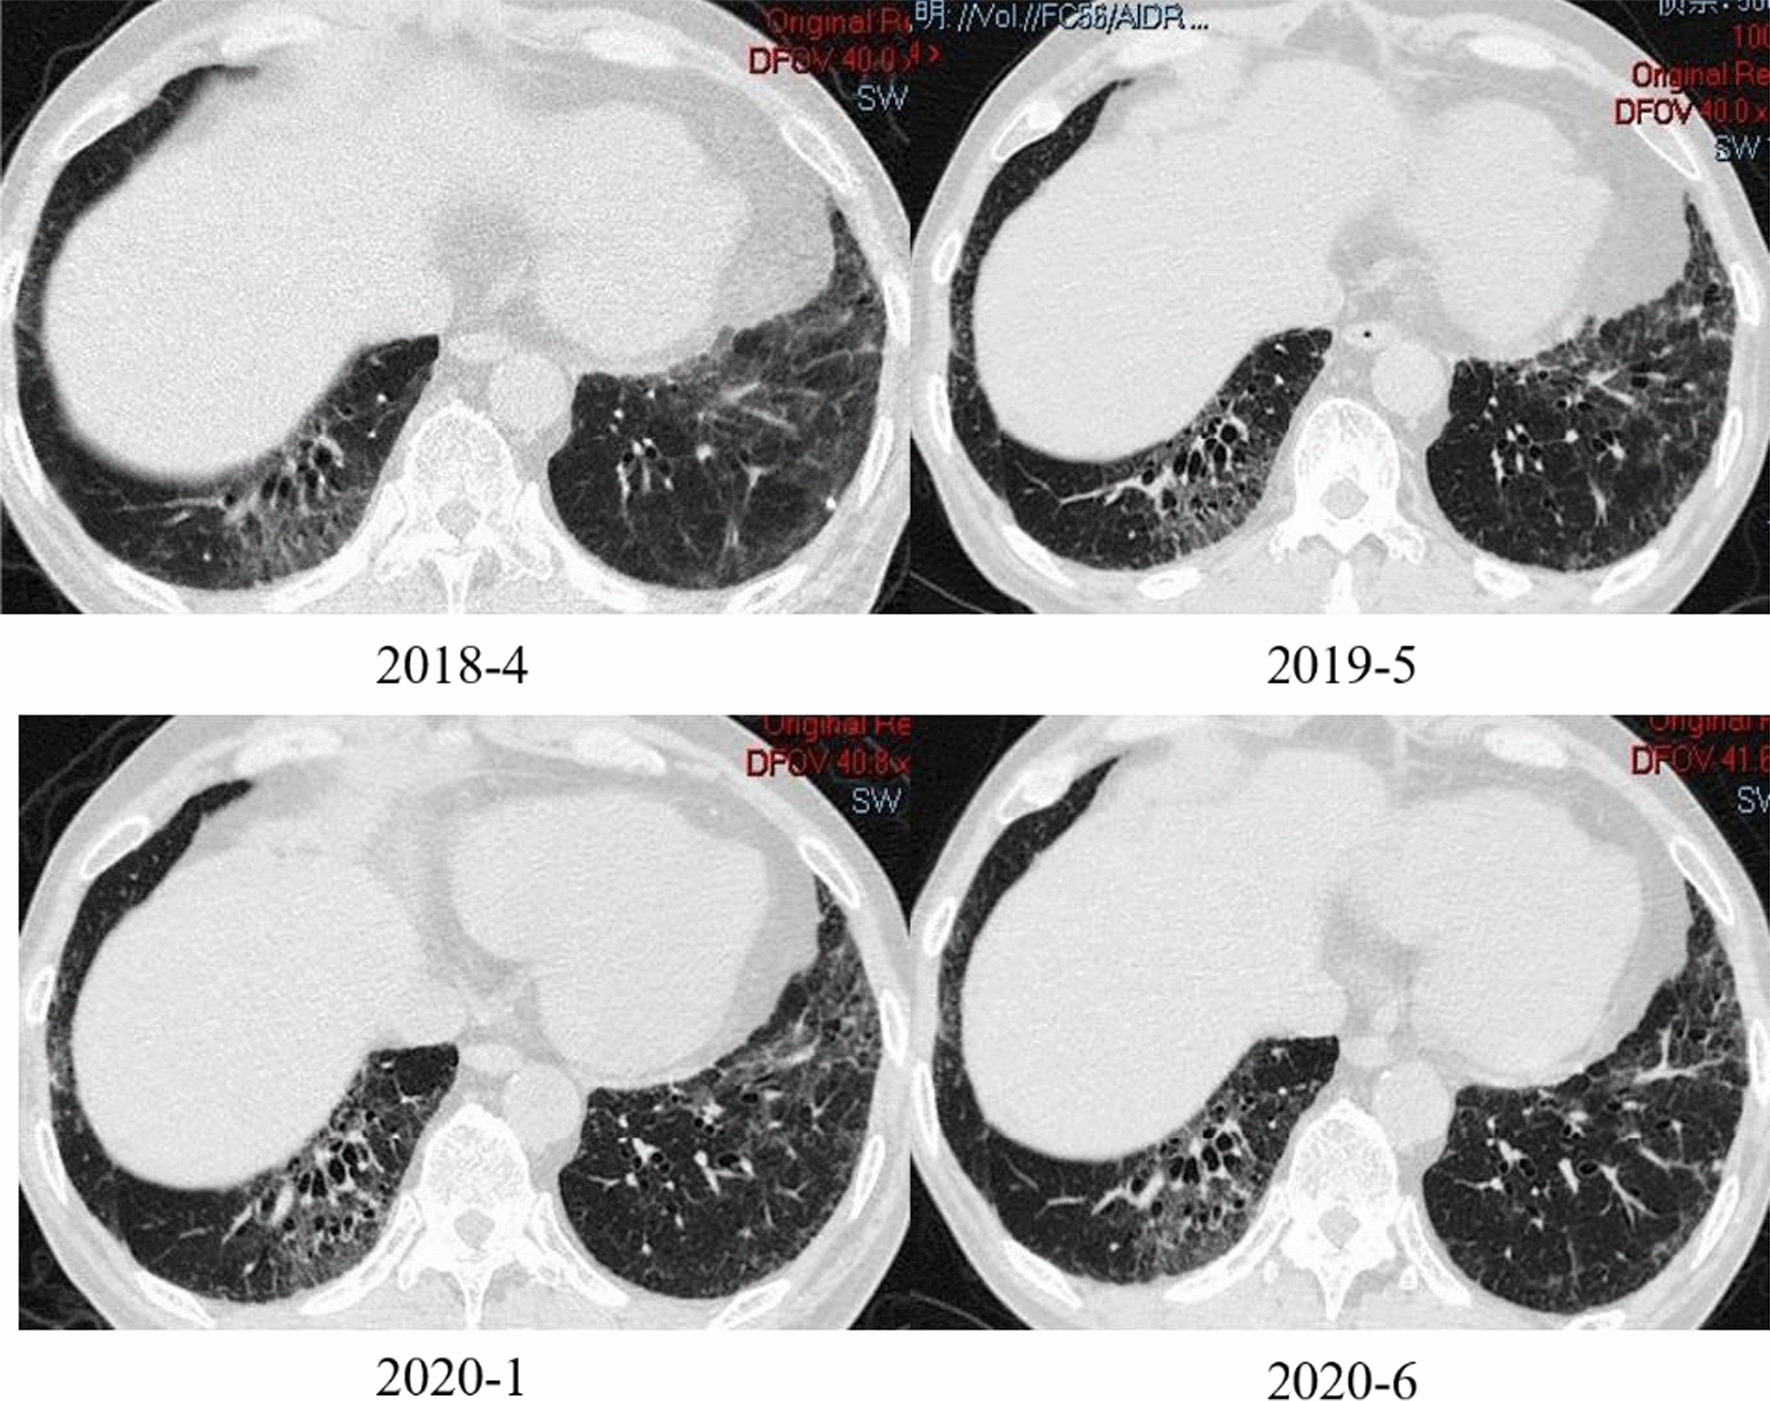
A 55-year-old male patient's lung CT, almost unchanged in 26 months
